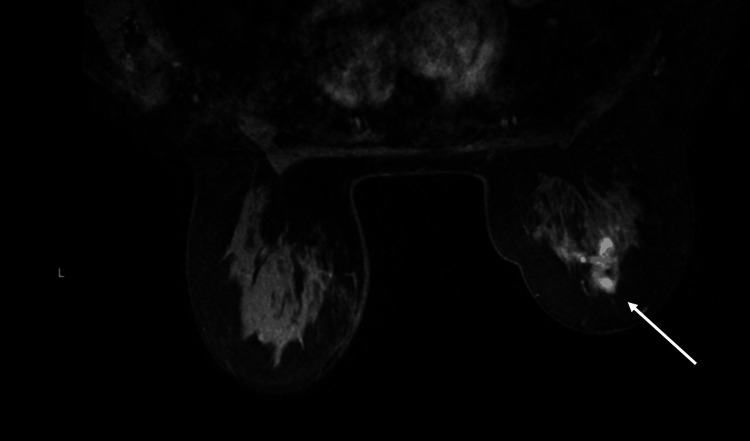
MRI from case one demonstrating a lesion in the right breast.
